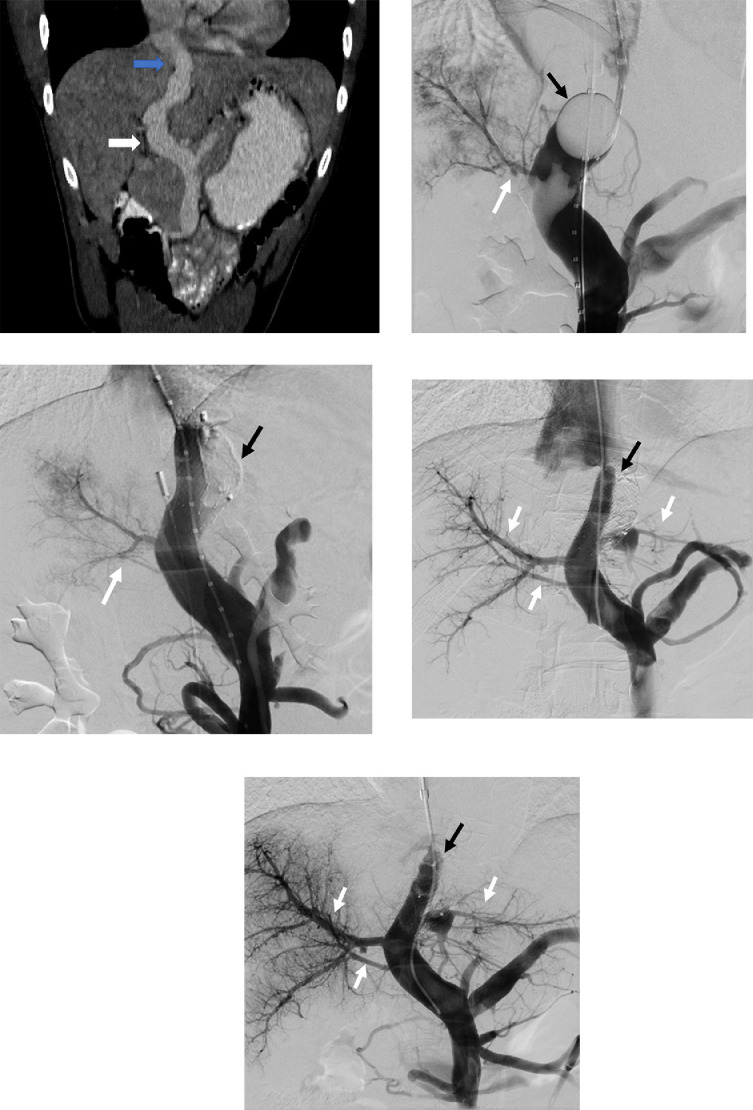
A 21-year-old male with a congenital extrahepatic portosystemic shunt (Abernethy malformation). (A) Findings: Coronal CT image obtained prior to the intervention demonstrates a large portosystemic shunt with the portal vein (white arrow) draining into the inferior vena cava via the middle hepatic vein (blue arrow), just before the cavo-atrial junction; (B) Balloon (black arrow) occlusion venogram before the first procedure shows a diminutive portal vein (white arrow); (C) Venogram following occlusion of one of the 2 parallel stents (black arrow) with slight improvement in portal vein size; (D) Venogram before the second procedure (performed 2 months after the first procedure) shows relatively developed intrahepatic portal veins (white arrows) with continued filling of the malformation via the patent stent (black arrow); (E) Venogram following occlusion of the second stent (black arrow) with significant improvement in portal vein filling (white arrows).
